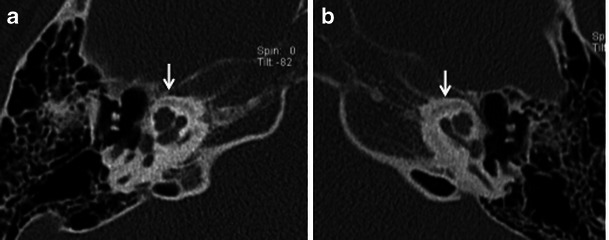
Axial HRCT images of the *right* (a) and *left* (b) temporal bone in a young adult with known osteogenesis imperfecta tarda and bilateral SNHL. Bilateral pericochlear ring-like hypodensity (*arrows*) closely mimics cochlear otosclerosis
